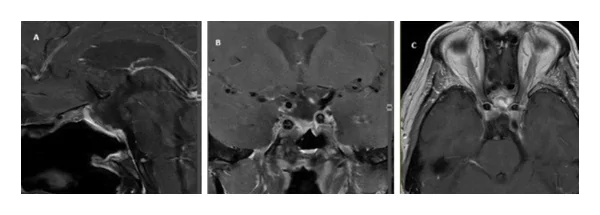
Postoperative MR images: (A–C) postoperative postcontrast T1‐weighted images demonstrate near‐total resection of the pituitary adenoma with decompression of the optic chiasm and floor of the third ventricle. Axial (A), coronal (B), and sagittal (C) views show minimal residual tumor within the left cavernous sinus.
